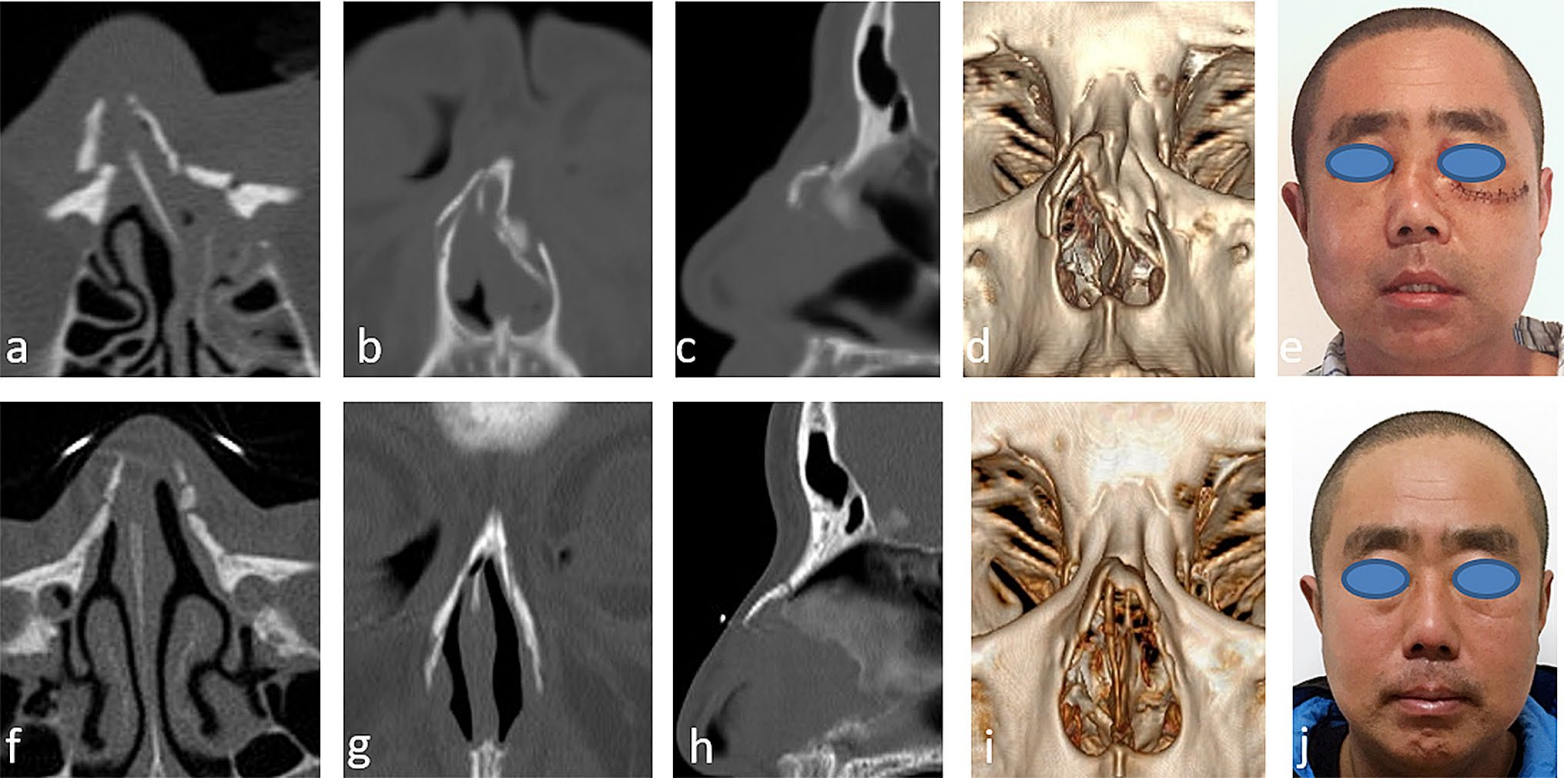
Images of a patient with nasal fractures. A 40-year-old male was knocked down by a heavy object resulting in nasal bridge deviation at work. After 7 days, he underwent EIIR and obtained satisfied aesthetics and good nasal airflow postoperatively. a Preoperative axial CT scan. b Preoperative coronal CT scan. c Preoperative sagittal CT scan. d Preoperative 3D CT scan. e Preoperative photo showing a depressed nasal bridge. f Postoperative axial CT scan. g Postoperative coronal CT scan. h Postoperative sagittal CT scan. i Postoperative 3D CT scan. j 6 month postoperative photo showing a well-corrected nasal bridge
